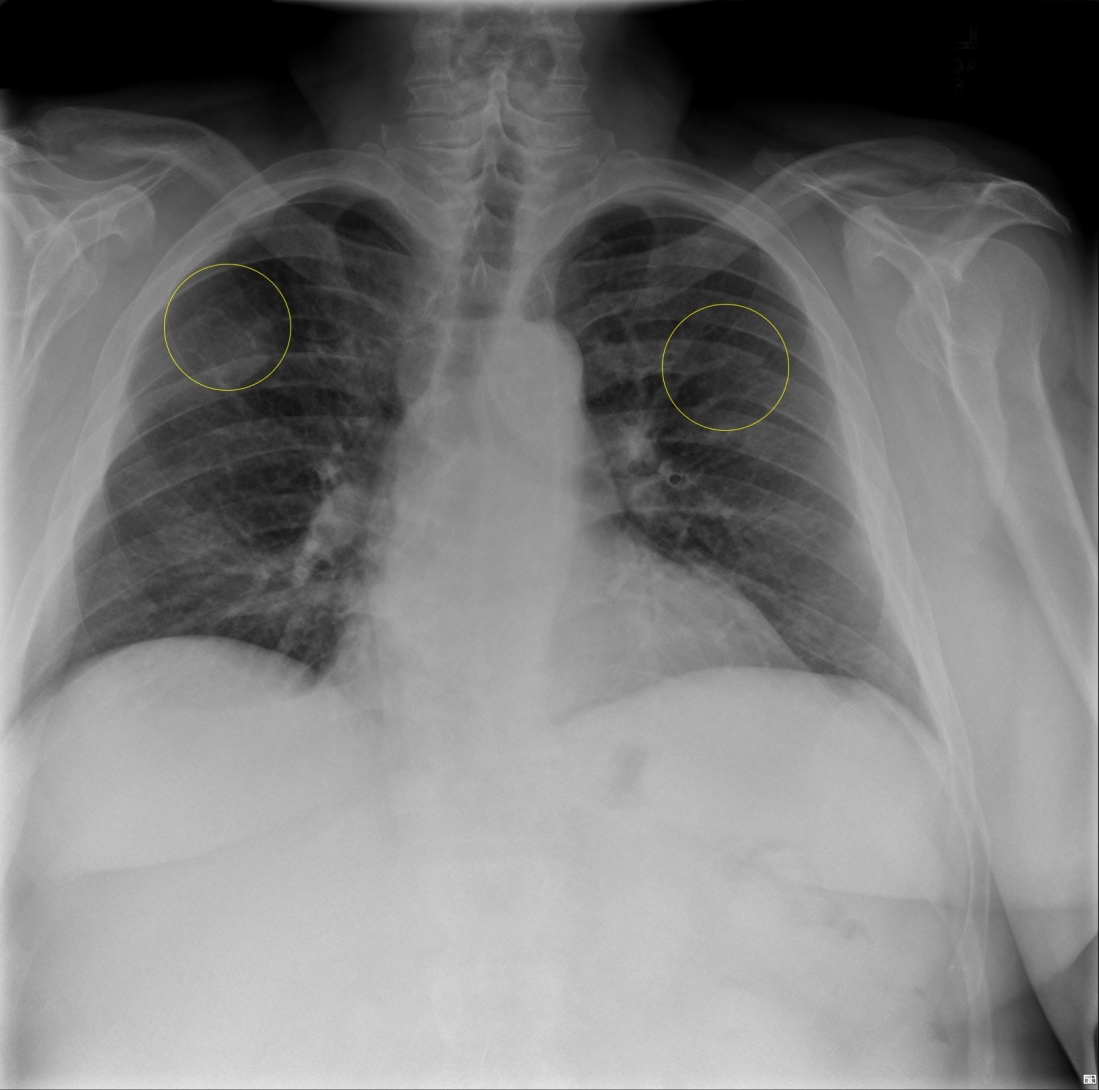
Chest x-ray showing multiple subacute rib fractures (yellow circles)
